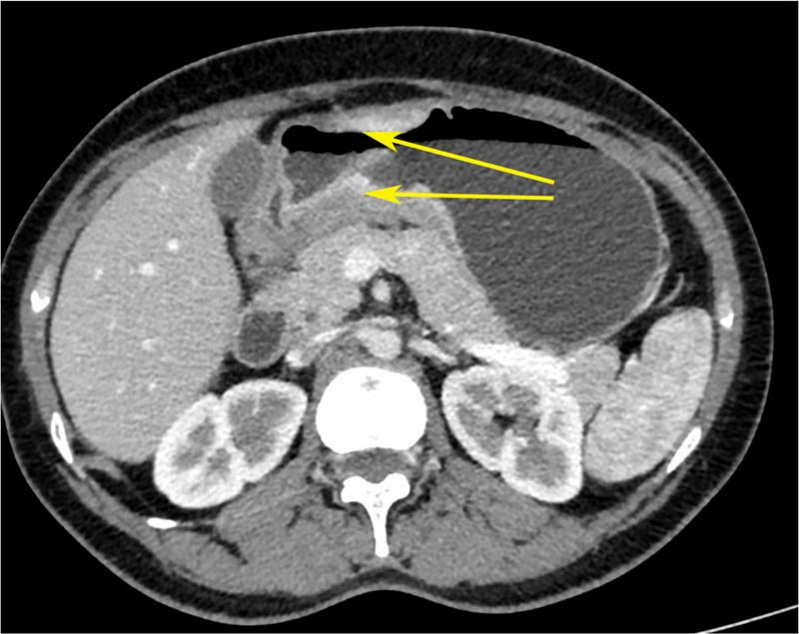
CT scan of the abdomen showing gastric wall thickening at the level of the pyloric antrum (yellow arrows).
